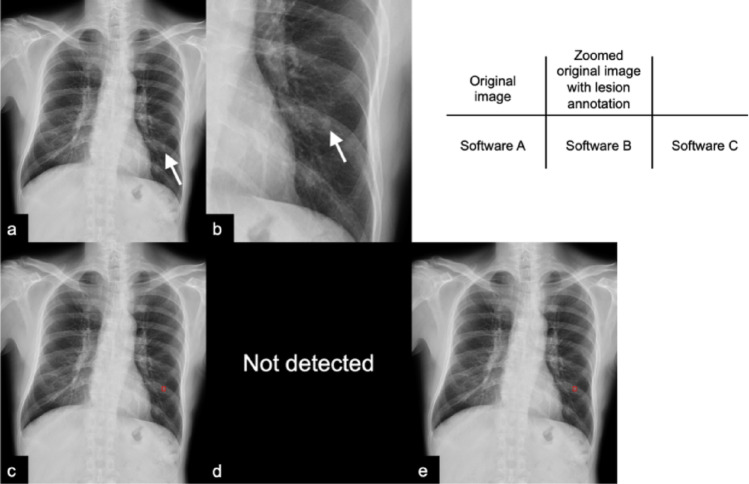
Representative case of the lesion missed by first reader but detected by two AI models (71-year-old, male, BI = 0). a and b chest radiograph of a lesion in left lower lung (arrow) detected by the second reader. c–e. The lesion was not detected by the first reader; in the AI outputs, Software A (c) and Software C (e) correctly localized the lesion (red bounding boxes), whereas Software B (d) produced no detection at the target site. The lesion’s visibility score was 2 (subtle)
